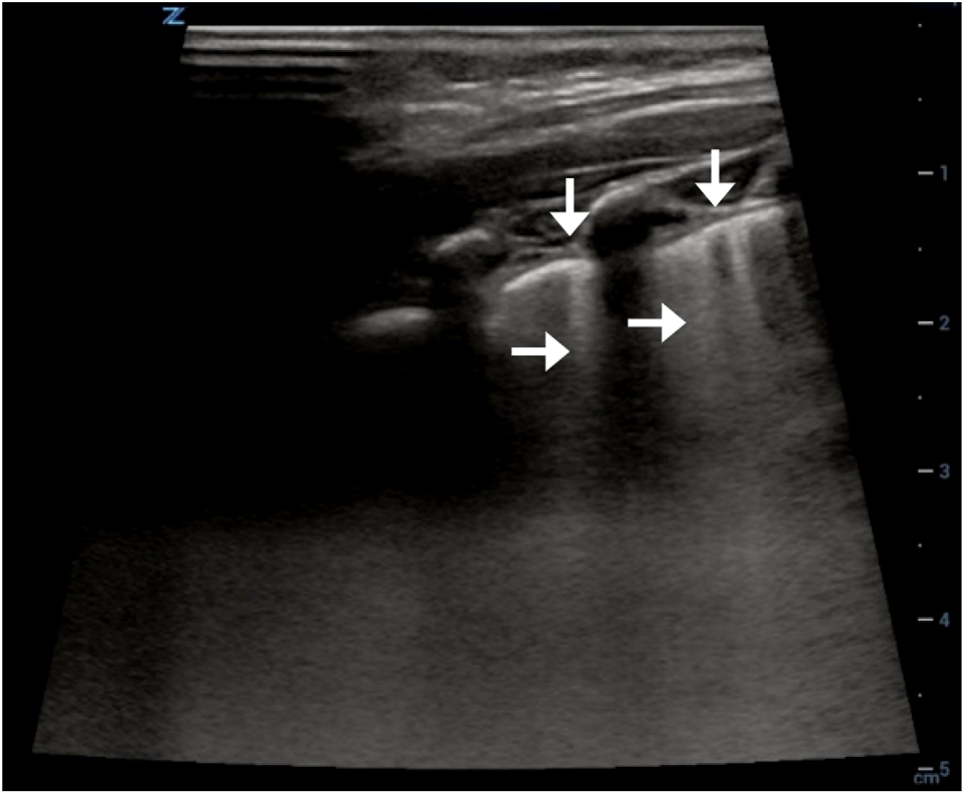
Focal pleural irregularities (vertical arrows) and B-lines (horizontal arrows) suggestive of pneumonia from right lung ultrasound performed on initial visit to the emergency department.
